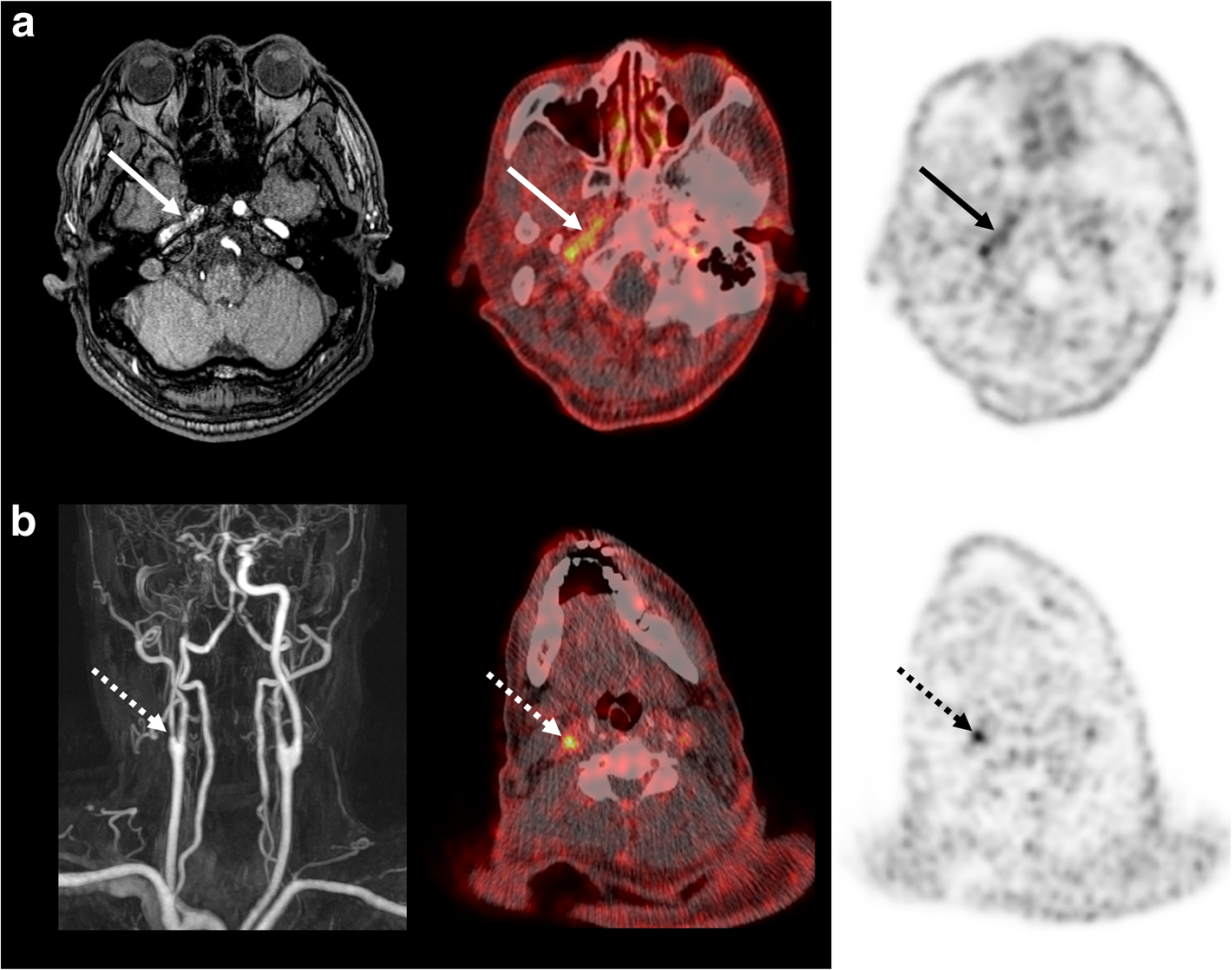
^18^F-GP1 PET/CT and magnetic resonance images of a 61-year-old man with acute cerebral infarction in the right middle cerebral artery territory and basal ganglia. Transaxial images of ^18^F-GP1 PET/CT at 60 min after injection show increased uptake in the petrous part of the right internal carotid artery (a, arrows) and right proximal internal carotid artery (b, dotted arrows). Magnetic resonance images reveal filling defects in right internal carotid artery (a, b). A smooth echogenic plaque was seen from the bilateral carotid bulbs to the proximal internal carotid arteries on transcranial Doppler (large-artery atherosclerosis subtype)
